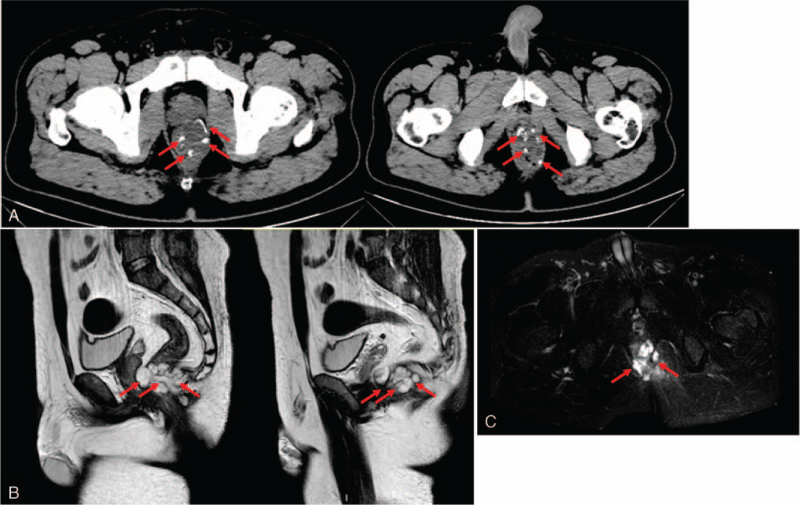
Imageological examination. (A) CT examination indicated the thickened wall of the lower rectum accompanied with a strip-shaped high-density shadow (red arrows). (B) MRI showed a cyst-like mass of about 33 × 57 × 30 mm in the anal area (red arrows). (C) MRI indicated plaque-shaped high signal shadow (red arrows) in the left side of the anus. CT = computer tomography, MRI = magnetic resonance imaging.
